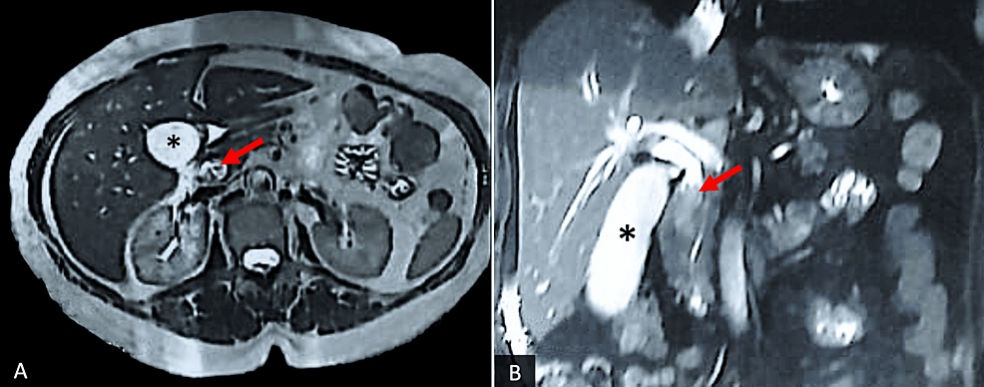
Axial abdominal MR T2-weighted imaging shows a dilated common bile duct (arrow), adjacent to the gallbladder (*) (A). Coronal MR T2-weighted imaging shows the gallbladder (*) with a thickened and dilated common bile duct upstream of a solid obstacle (arrow) (B).
